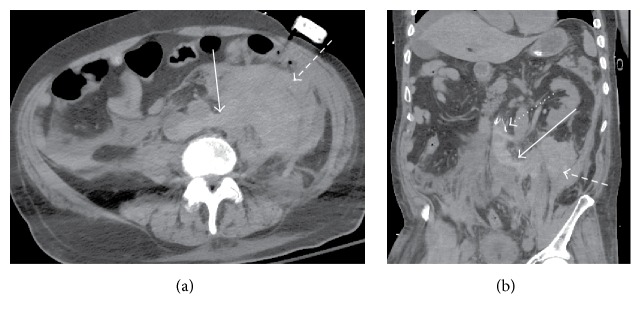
(a) Initial noncontrast CT axial image demonstrated a large high density left retroperitoneal hematoma (dashed arrow) contiguous with left site of aorta and IVC (arrow). (b) Initial noncontrast CT coronal reformatted images demonstrated a large high density left retroperitoneal hematoma (dashed arrow) contiguous with high-attenuated tubular structure arising from the left side of IVC (arrow), likely a lumbar vein. There is an expansile appearance of infrarenal IVC. IVC filter above the left inferior renal vein is also appreciated (dotted arrow).
